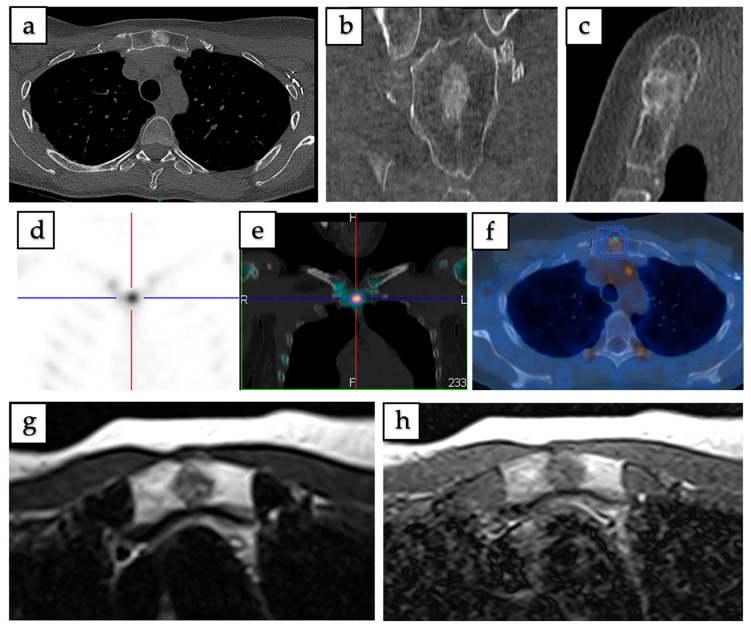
Imaging of intraosseous hibernoma confirmed by biopsy in a 49-year-old female involving the manubrium sterni. (a–c) Axial, coronal, and sagittal CT scans demonstrate a well-defined 13 mm sclerotic lesion. (d,e) SPECT-CT reveals increased radiotracer uptake localized to the lesion. (f) ^18^F-FDG PET/CT shows mild metabolic uptake with a maximum standardized uptake value (SUVmax = 3.2). (g) Axial T1-weighted MRI demonstrates intermediate signal intensity. (h) Axial T2-weighted MRI demonstrates a heterogeneous lesion with mild hyperintensity.
